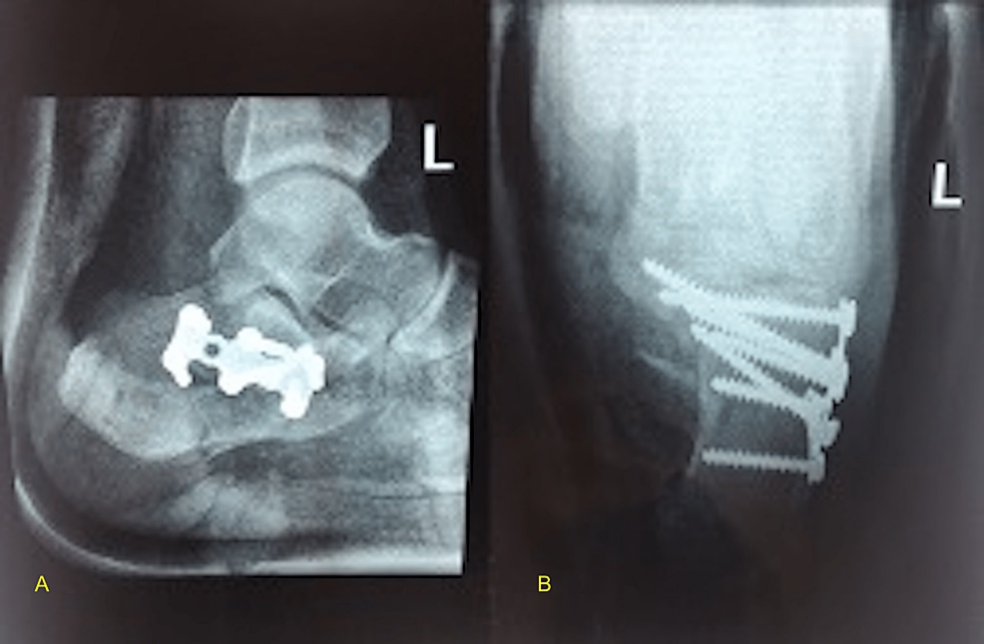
Postoperative radiographs Postoperative radiographs of the same 31-year-old gentleman after undergoing open reduction internal fixation of the right calcaneus fracture with the distracted prone setup demonstrating appropriate reduction and restoration of joint surface. A) Lateral view; B) axial view
